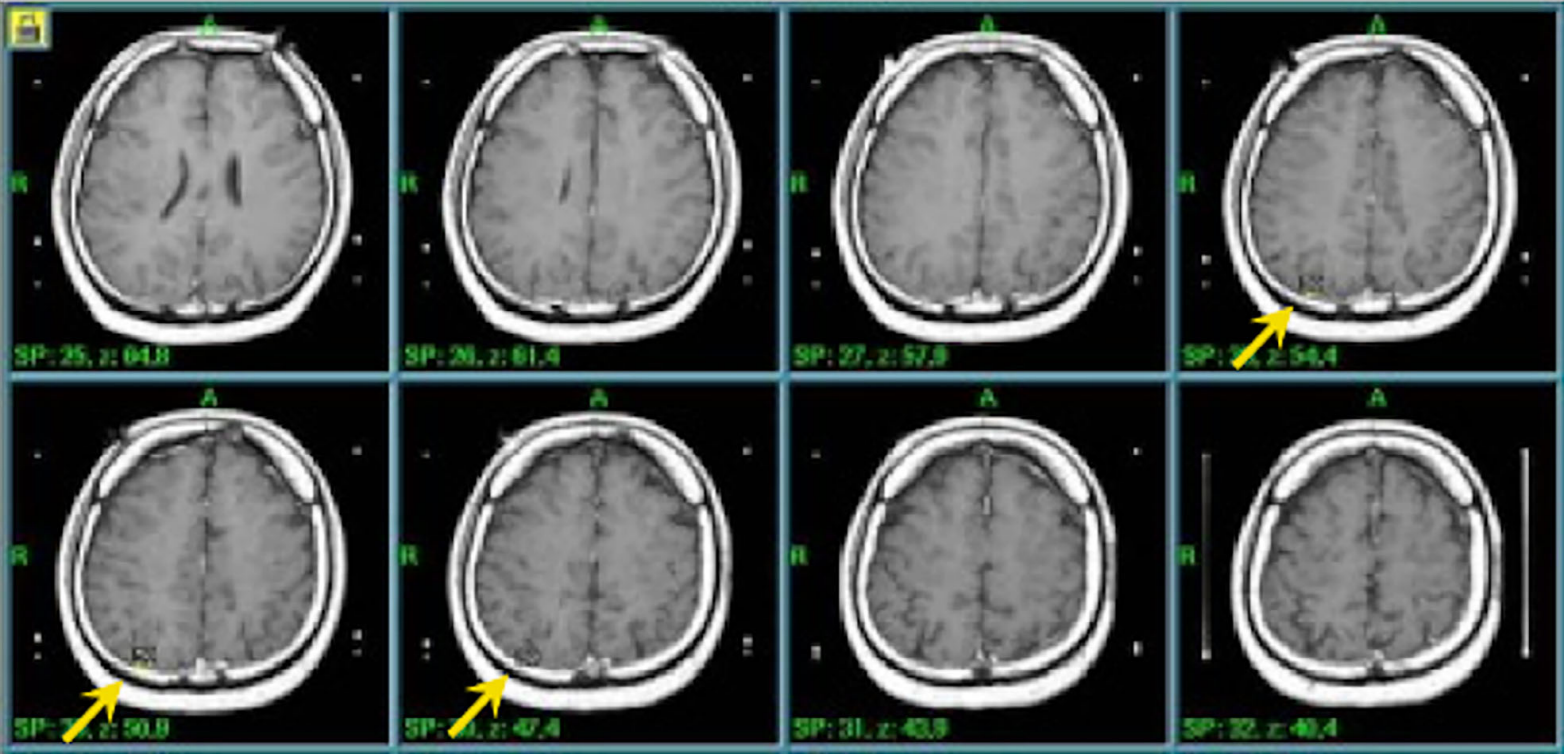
Planning computed tomography (CT) images of stereotactic radiotherapy with isodose distributions.
